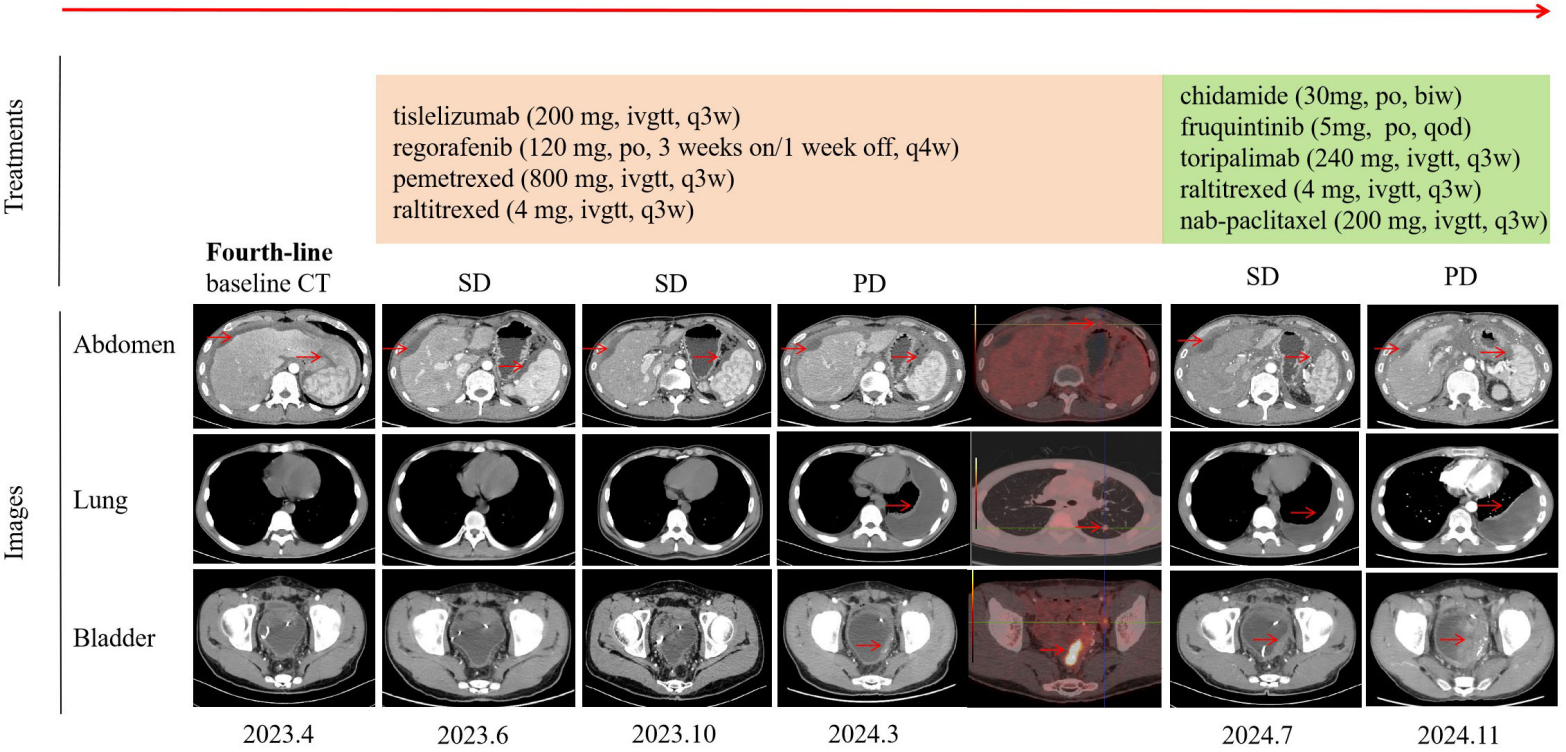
Timeline of treatment and efficacy evaluation. The “Treatments” section detailed the timeline of therapeutic regimens administered. The “Images” section included chest, abdomen, and pelvis CT scans, as well as positron emission tomography images at various stages of disease progression. Efficacy was evaluated according to the RECIST criteria. Tumor lesions are highlighted by red arrows. CT, computed tomography; SD, stable disease; PD, progression disease.
